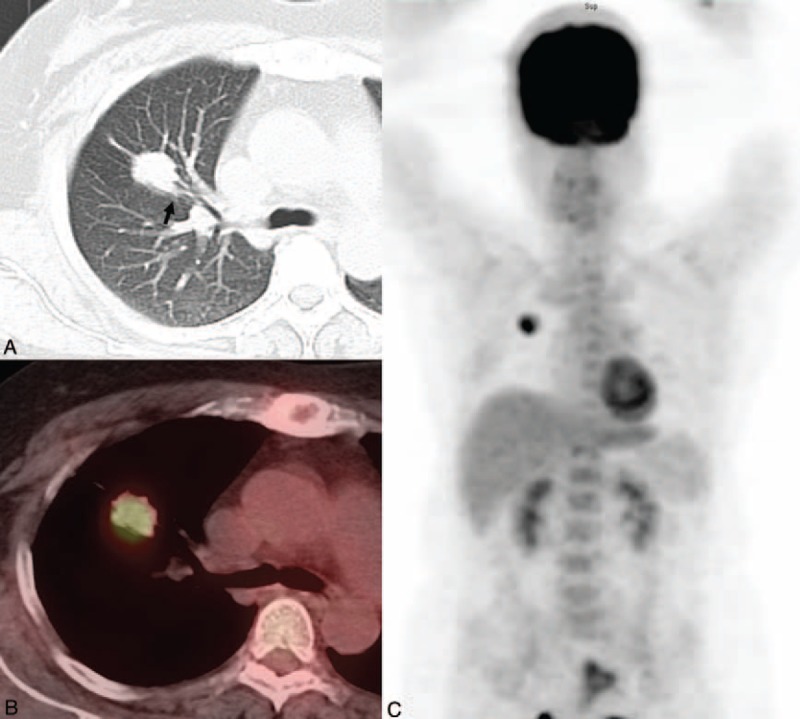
Representative images of a 50-year-old female with a pathological stage IA adenocarcinoma. (A) Lung window of CT scan showing a 2.6-cm lobulated solid tumor with a thickening bronchovascular bundle (arrow) in the right upper lobe. ^18^F-FDG PET/CT in the axial plane (B) and whole-body maximum-intensity-projection (C) images demonstrating high ^18^F-FDG avidity (SUVmax, 5.6) in the primary lesion. The patient encountered tumor recurrence during a 16-month follow-up. CT = computed tomography, FDG = fluorodeoxyglucose, PET = positron emission tomography, SUVmax = maximal standard uptake value.
